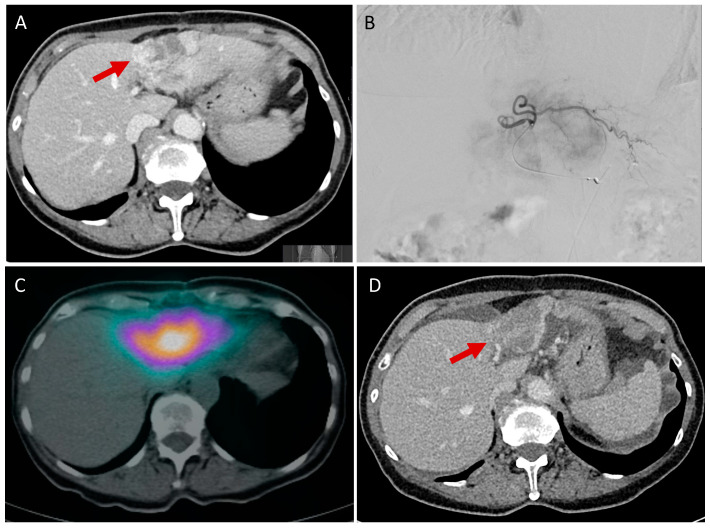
Example of intrahepatic CCA in liver segments II and III with vital tumor portions, as visible by the hypervascularized peripheral tumor parts (A, red arrow). After selective internal radiotherapy using 0.44 GBq ^90^Yttrium-loaded particles by catheter positioning in the left hepatic artery (B) and acquisition of a SPECT/CT (C), postinterventional follow-up 6 weeks after SIRT showed a marked decrease in vital tumor parts (D, red arrow).
